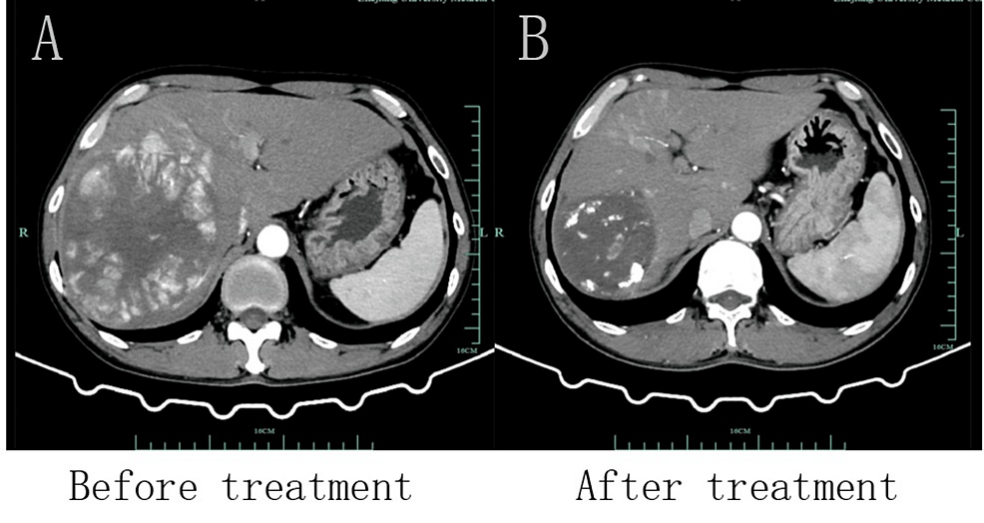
After TACE treatment with targeted immunotherapy, the patient's liver lesions reduced significantly. A. CTA image of the liver before treatment; B. CTA image of the liver after treatment. TACE: transarterial chemoembolization; CTA: computed tomography angiography
